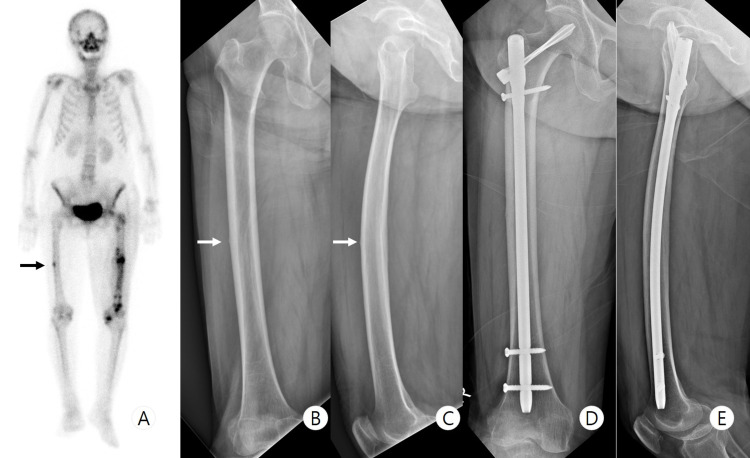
Previous preoperative imaging and six-month follow-up X-ray - case 1 Preoperative bone scan (A) shows hot uptake at the right femur midshaft. White arrow indicates cortical hypertrophy and radiolucent line on preoperative anteroposterior (B) and lateral (C) radiographs. The six-month follow-up X-ray images show the disappearance of the radiolucent lint and the decrease in cortical hypertrophy (D, E)
